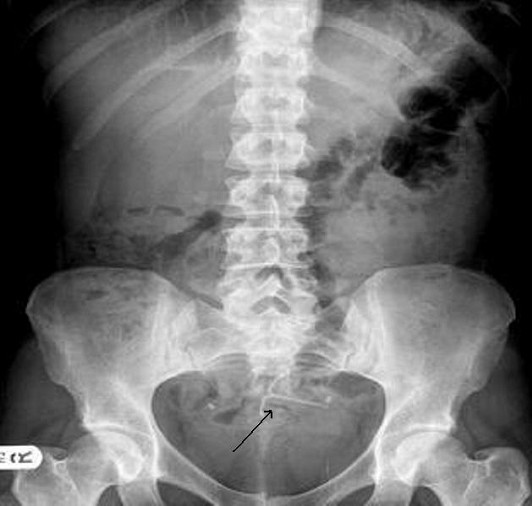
Abdominal X-ray with the Mirena IUS (arrowed).
